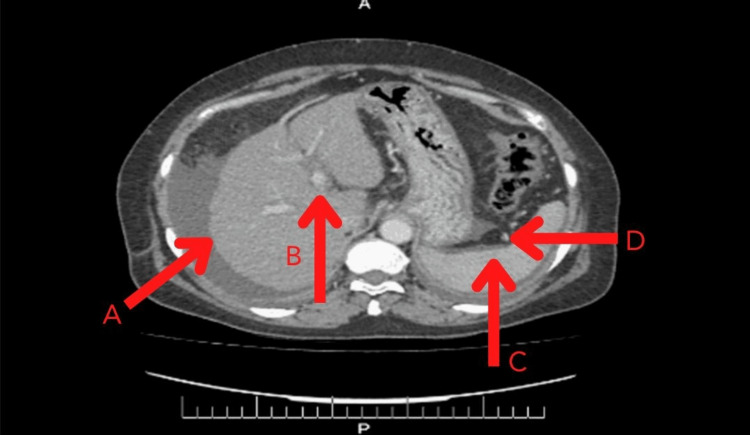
CT of the abdomen and pelvis The liver was subjectively small in size and showed serrated margins (A). The portal vein was mildly dilated and measured approximately 1.3 cm (B). The spleen was mildly enlarged with a splenic index of 550 (C). Few tortuous venous channels were seen at the splenic hilum (splenic varices; D).
